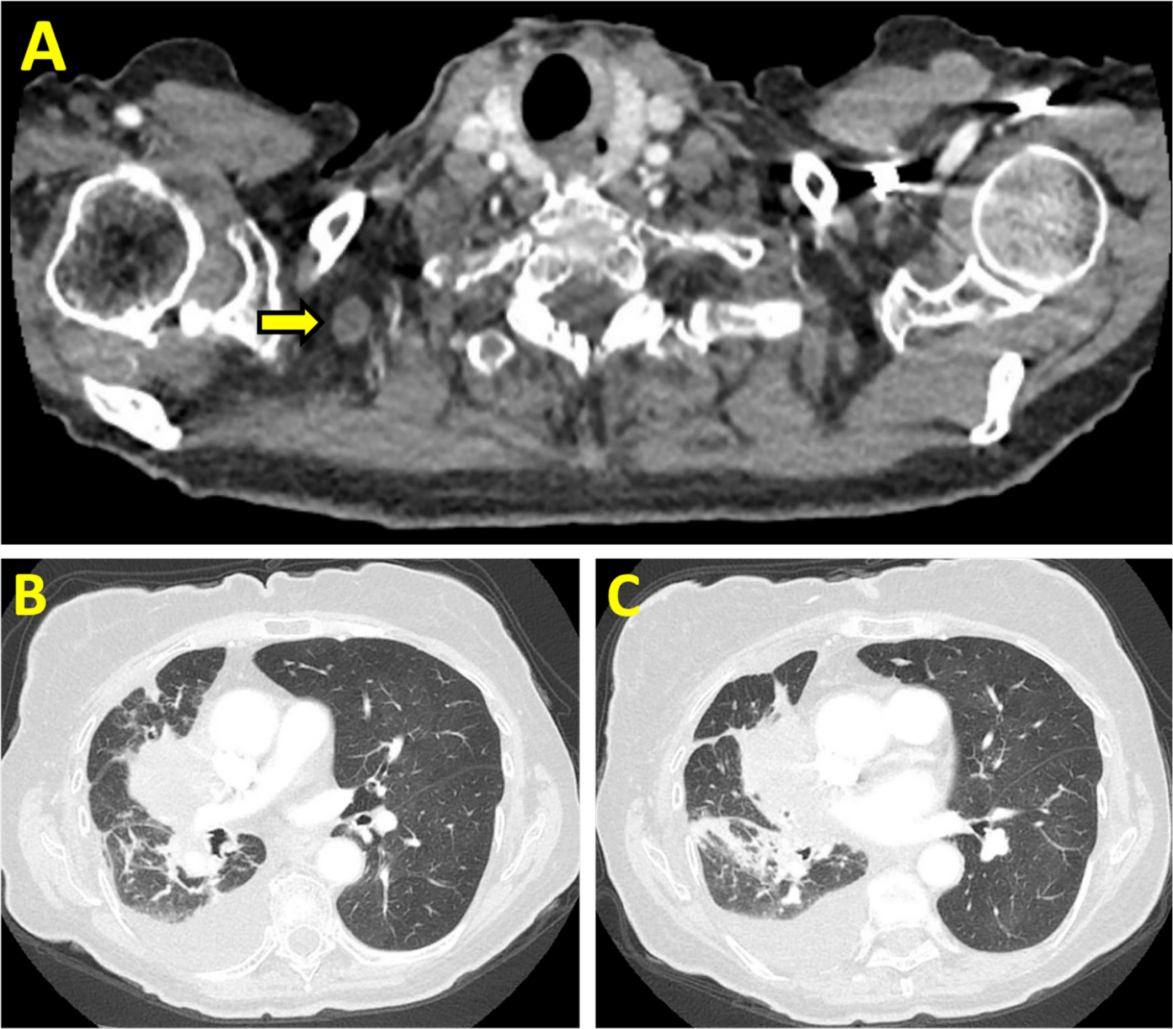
Axial CT chest images: (A) reduced size of right supraclavicular lymph node (arrow); (B and C) decrease in size of right lung mass.
